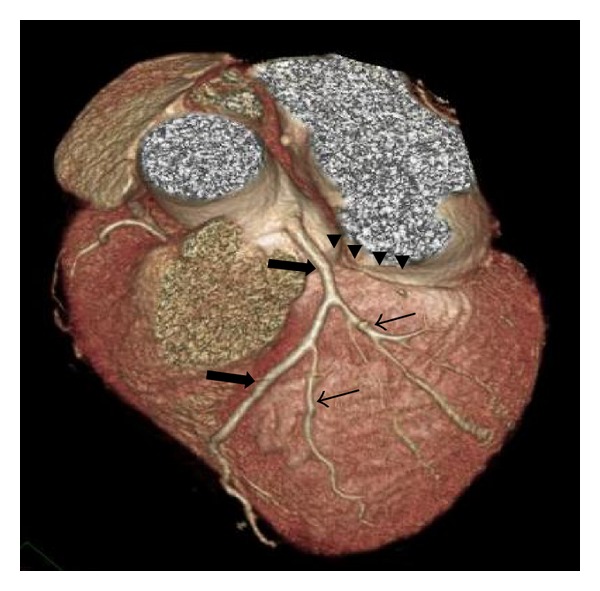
Top view three-dimensional computed tomography scan shows absent left circumflex artery in the atrioventricular groove (arrowed heads) with single left anterior descending artery (thick arrows) and prominent diagonal branches (thin arrows).
